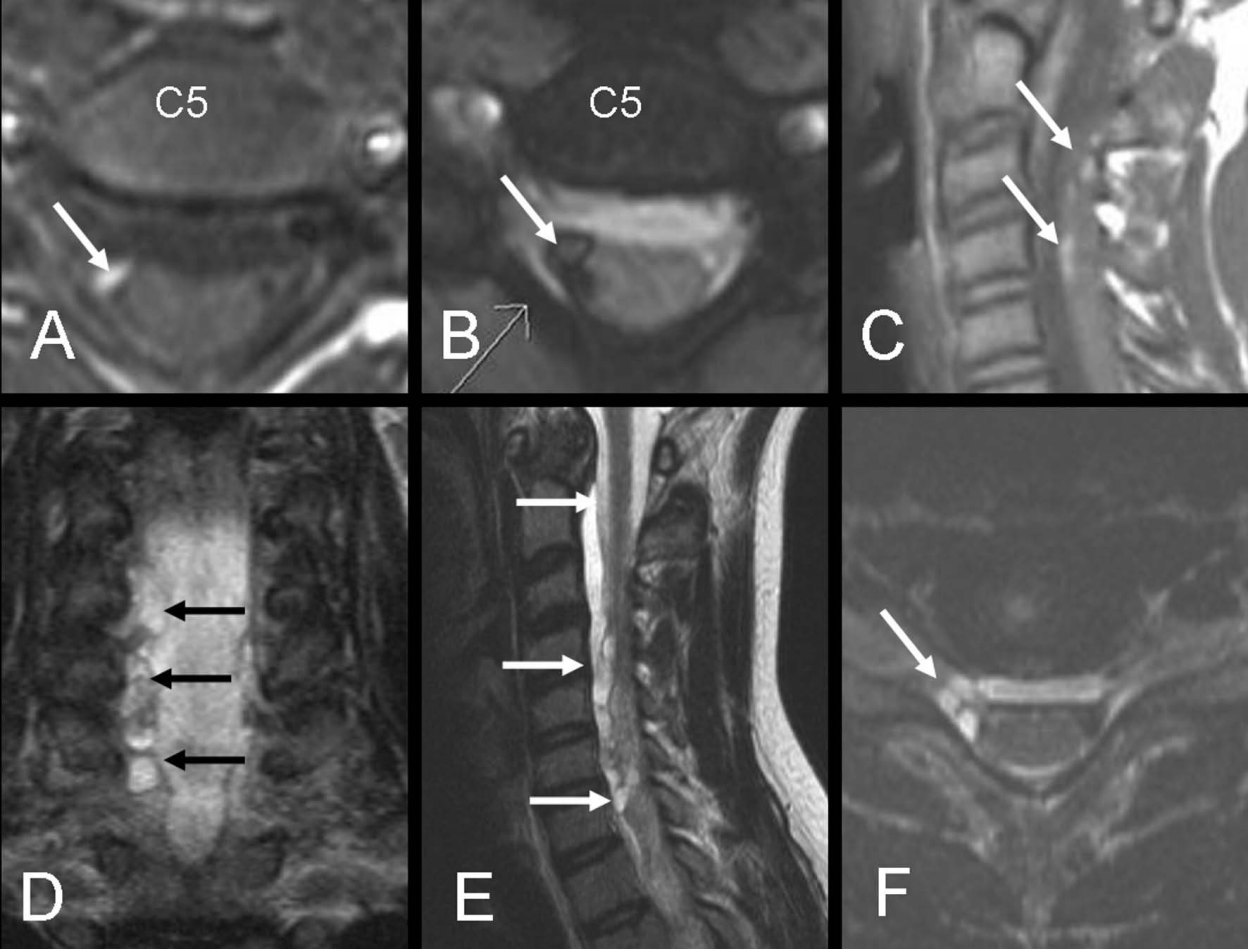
(A-B) axial T1WI and turbo flash image. (C) sagittal T1WI of the initial MRI of patient No 5 show methemoglobin at the C5 root exit with high signal intensity on T1WI and extremely low signal intensity on turbo flash images (arrows). Prior to surgery a new MRI (D-F) coronal STIR, sagittal T2WI and axial turbo flash image showed development of pseudomeningocele (intradural cysts) along the nerve roots at several levels (arrows). Despite these findings the roots were found to be intact (axonotmesis) on exploration (false positive MRI-finding).
